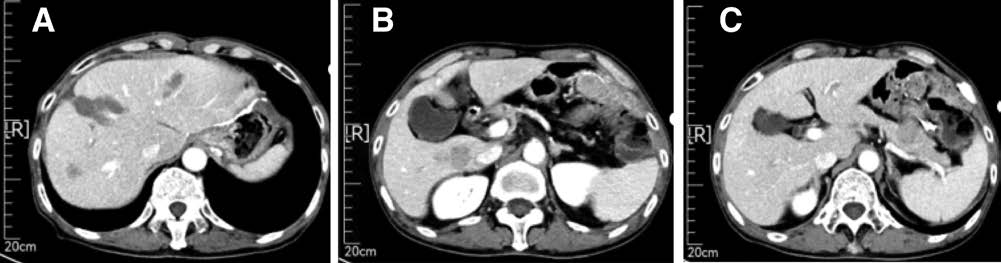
CT (2023-1-3): Compared with CT on September 14, 2022, nodules in the S7 and S6 segments of the liver increased; enlarged nodules at the junction of the pancreatic body and tail; there is a small amount of fluid accumulation around the liver, and there is no significant change in the rest. CT = computer tomography.
